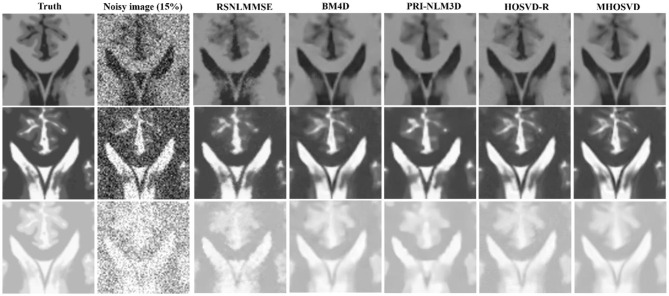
Performance comparison with enlarged region of interest (ROI) visualization using four different methods under 15% Rician noise. ROIs selected from above (red rectangles in Figures 2–4). First line: T1-weighted (T1w) images. Second line: T2-weighted (T2w) images. Third line: proton density-weighted (PDw) images.
